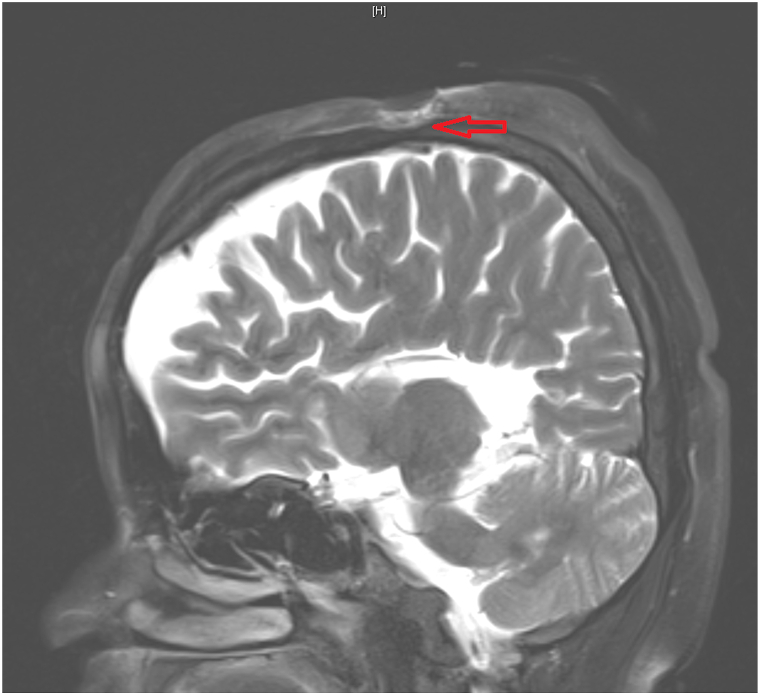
Cranial magnetic resonance imaging showing a deep vertex scalp ulcer (red arrow) extending to and involving the calvarial outer table.
